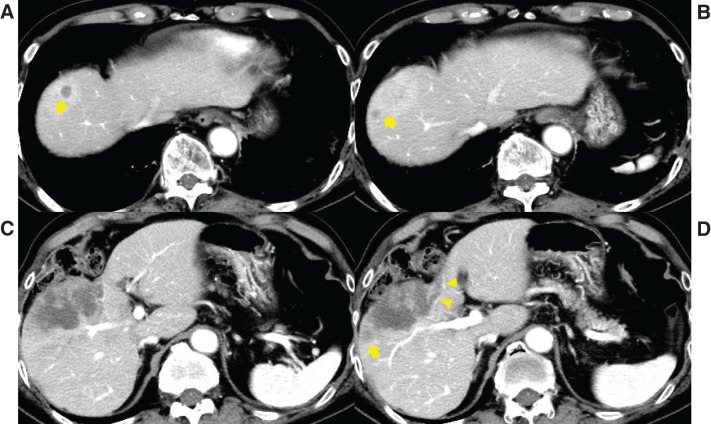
Preoperative CT images of the liver detected a tumor 80 mm in diameter located mainly in the right anterior section (C). Small nodules surrounded the main tumor (A, B, D). Extension of thrombus in segment 4 of the portal vein was apparent (D). No obvious lymphadenopathy was observed. Arrows indicate likely small intrahepatic metastases. Arrowheads indicate tumor thrombus in the portal vein.
